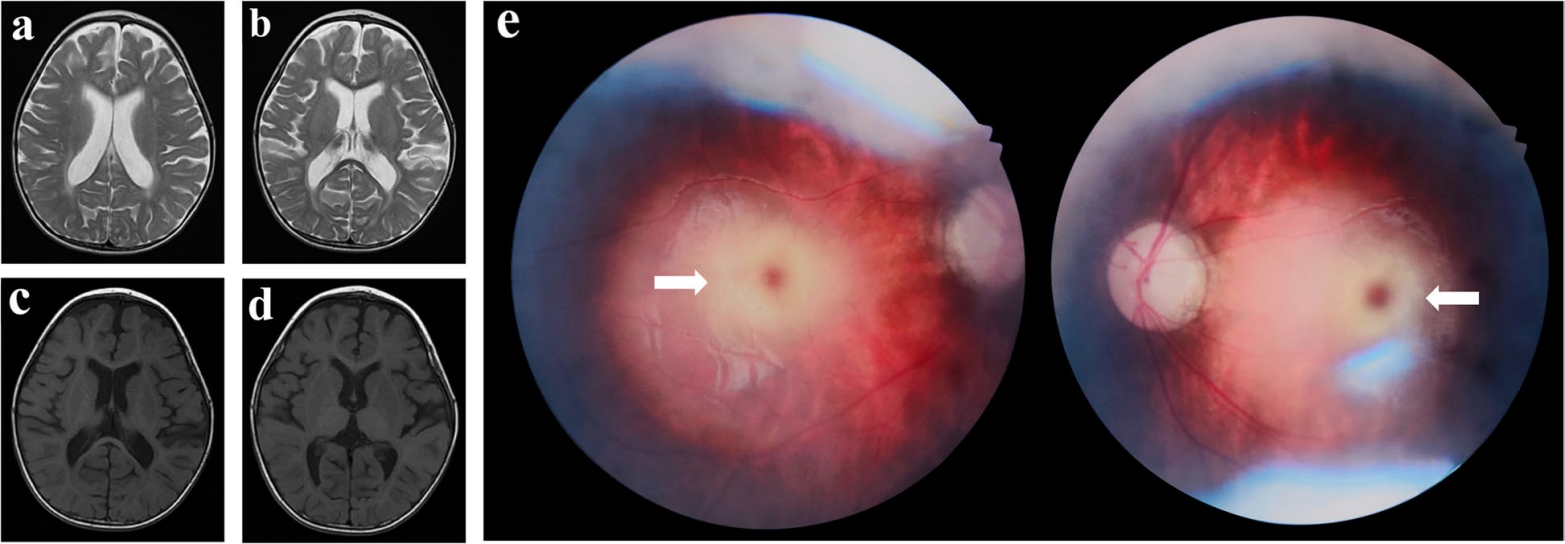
Cranial MRI of the case at age 22 months. a, b T2 weighted images; c, d T1 weighted images. Multiple symmetrical patches of slightly longer T1 and slightly longer T2 signals were seen in the white matter of the bilateral cerebral hemispheres and bilateral basal ganglia nuclei. Myelination of white matter is delayed. The corpus callosum is slender. The ventricular system, sulcus, fissure and pool are widened. e Fundus film of the case. The borders of the optic discs of both eyes were clear and pale, and the vascular pathways were acceptable, with cherry red spots visible in the macula. (white arrows)
